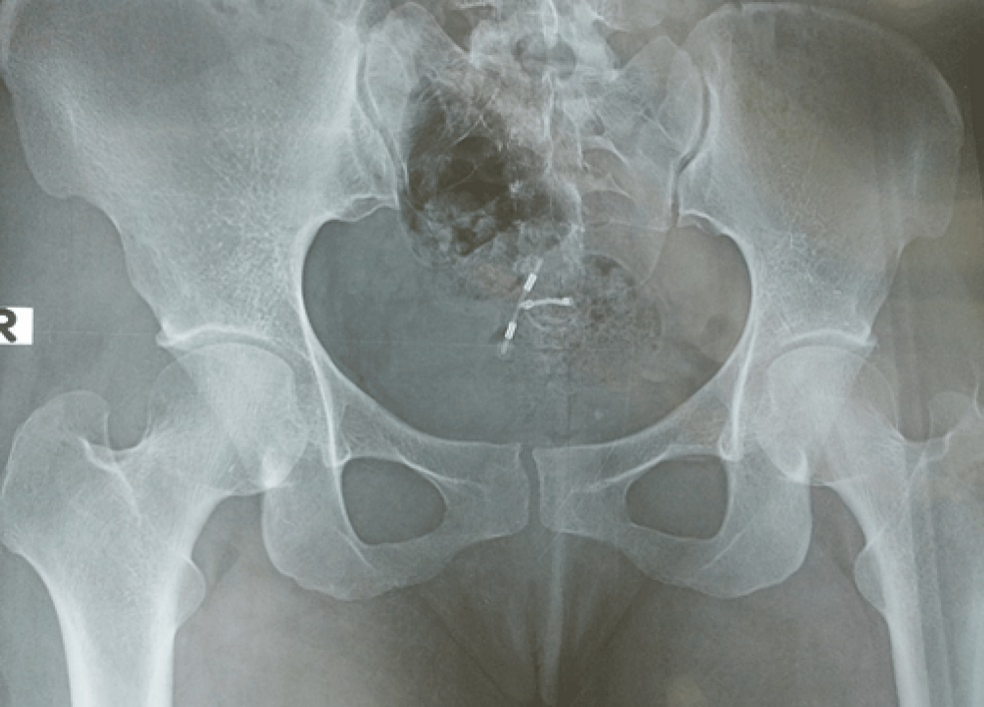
Plain AP X-ray showing IUCD in the pelvis but with malposition (note the halo around the lower arm of the IUCD representing shadow from the urinary stone) AP: anteroposterior; IUCD: intrauterine contraceptive device
